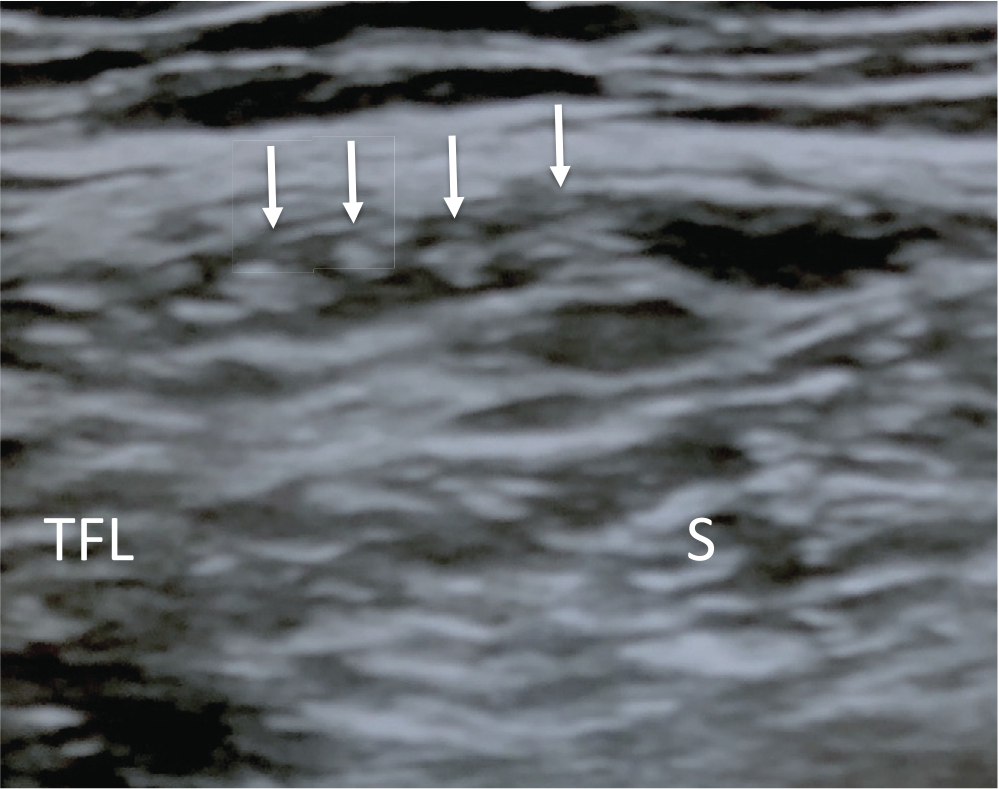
Transverse ultrasound image of the Fan type within the intermuscular space between the sartoirus and the tensor fascia latae. S: Sartorius, TFL: tensor fascia latae muscle, Arrows: several nerve branches.
